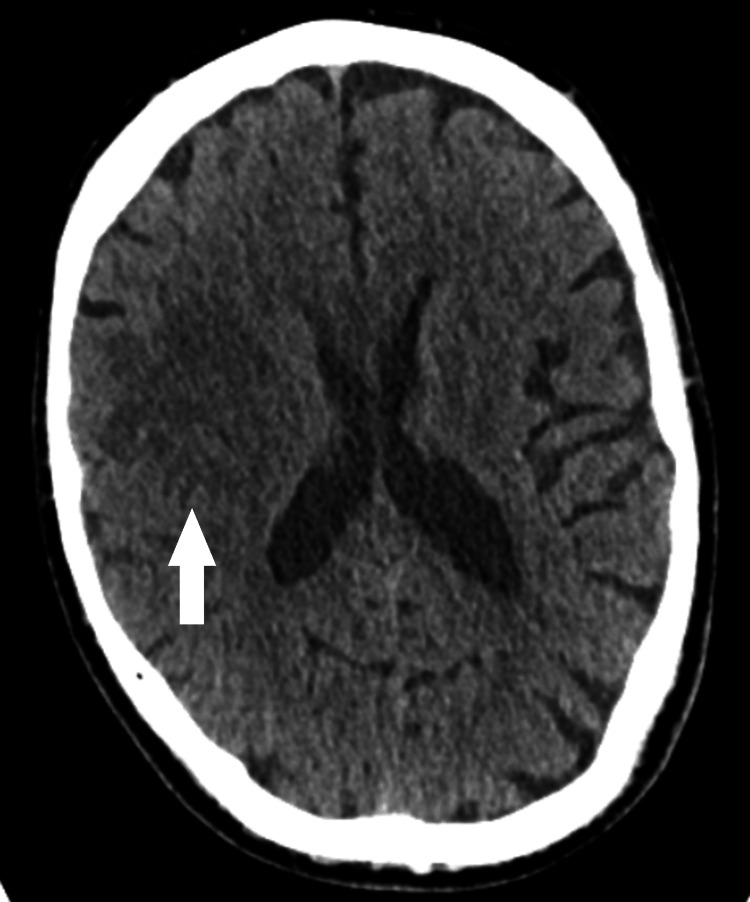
CT scan of the head A hypointensity in the right hemisphere was noted, which was not conclusive in terms of the underlying pathology CT: computed tomography
